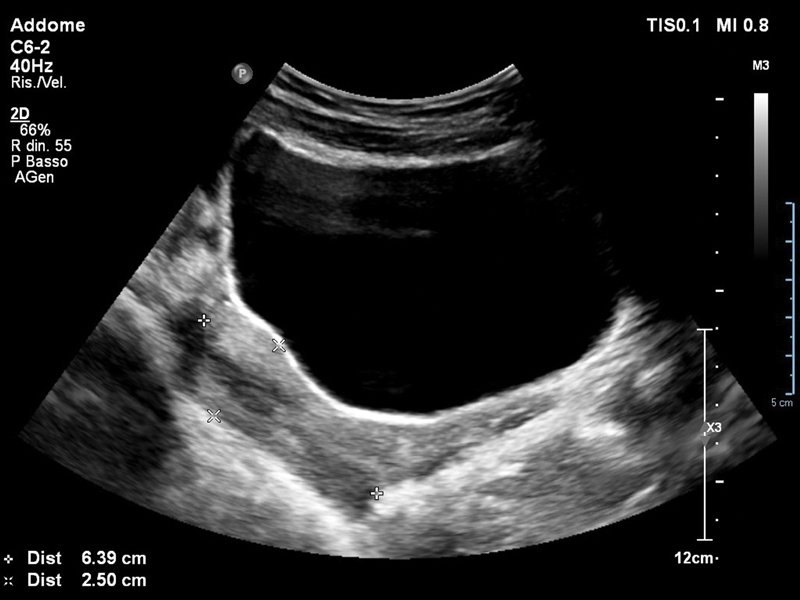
Normal uterus on ultrasound after 12 months.
